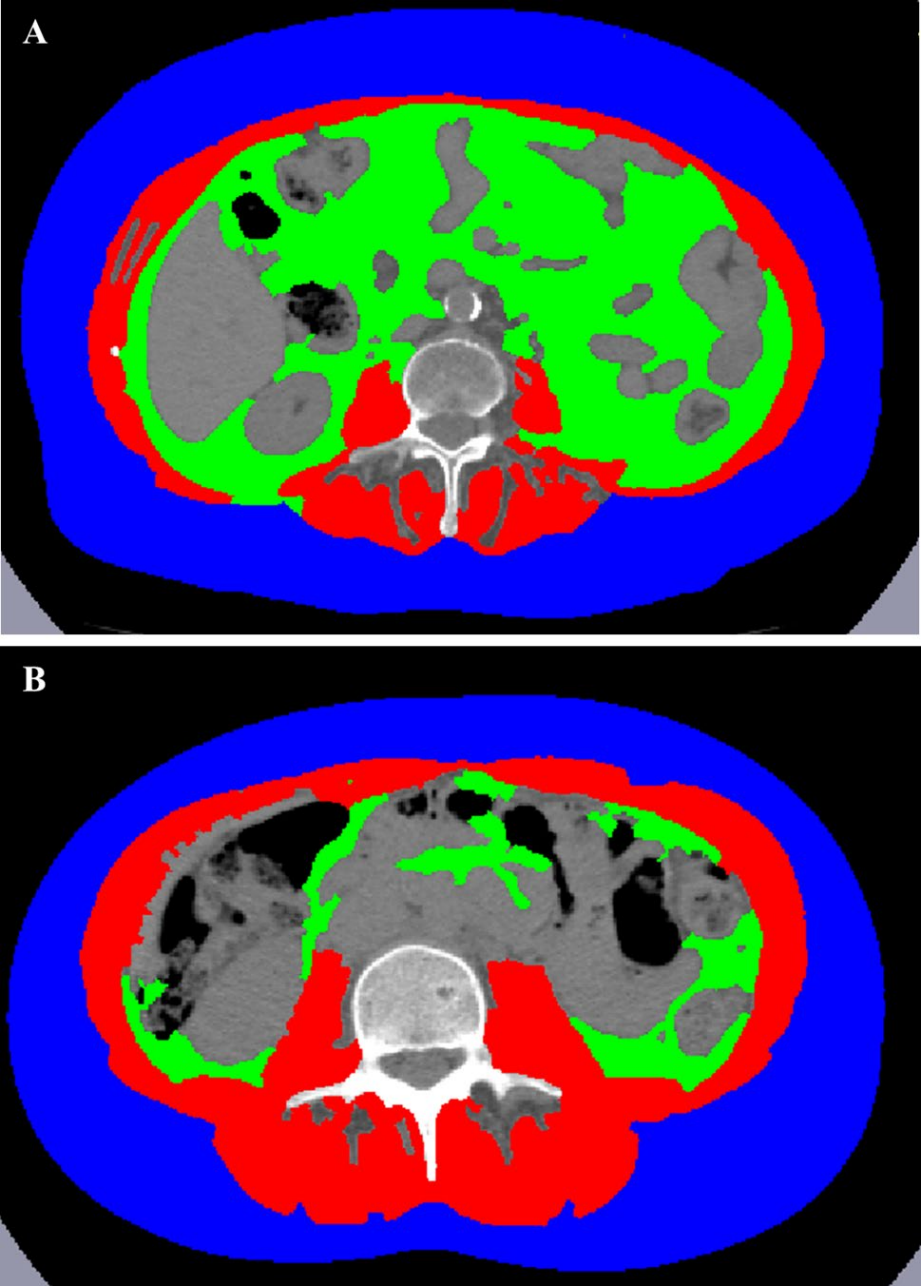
Body composition on computed tomography images of the same sarcopenic (image A) and non-sarcopenic (image B) patients of Fig. 2. Skeletal muscle area is shown in red colour, visceral adipose tissue area in green and subcutaneous adipose tissue in blue
